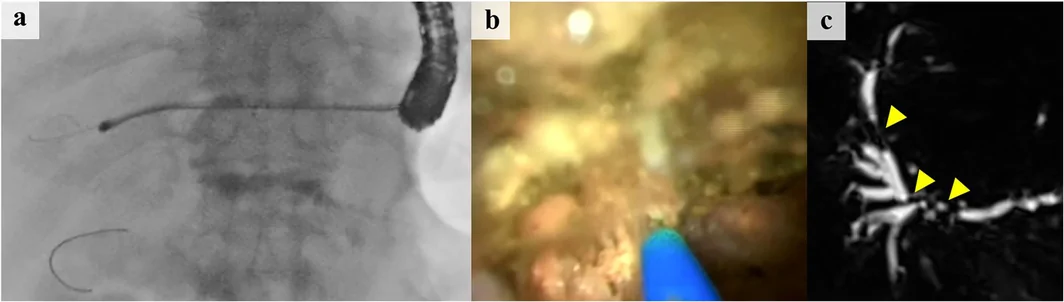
(a) Fluoroscopic image shows a slim cholangioscope advanced into the right intrahepatic bile duct via the endoscopic ultrasound–guided created route. (b) The cholangioscopic view during long‐pulse laser lithotripsy shows fine stone fragmentation. (c) Magnetic resonance cholangiopancreatography shows multiple stones in the right intrahepatic bile duct (yellow arrowheads).
